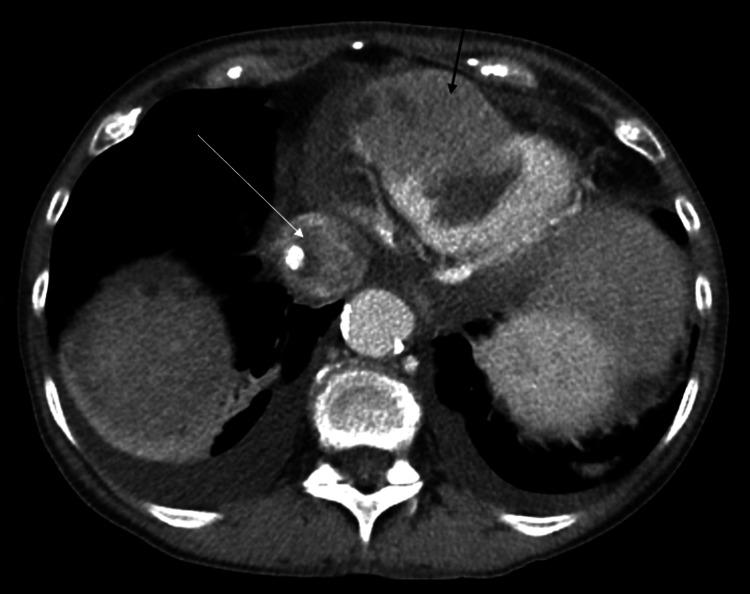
CT Abdomen with contrast in the transverse plane showing the cardiac mass with mixed attenuation in the right ventricle (black arrow) as well as within the inferior vena cava (white arrow).
